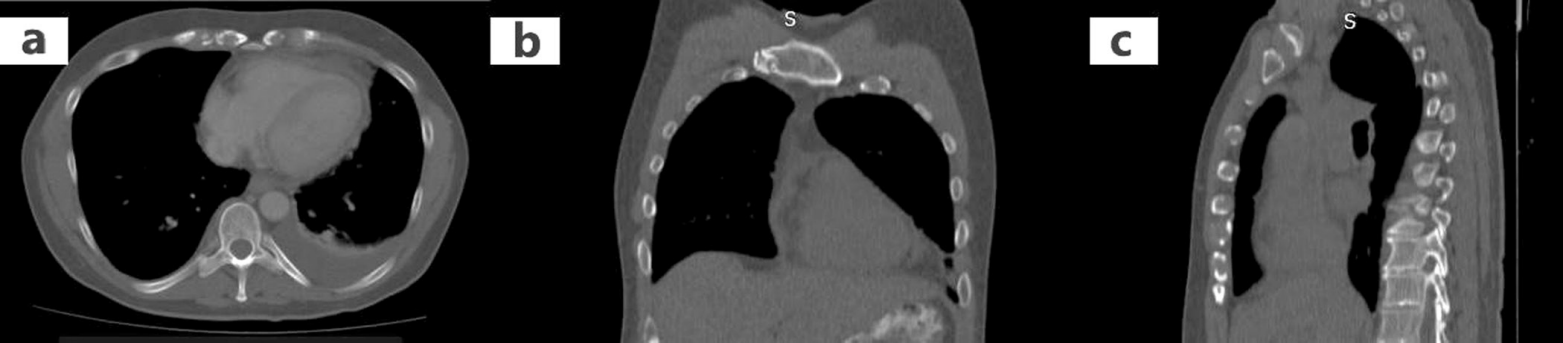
Chest computed tomography (CT) revealing a pericardial effusion and a small left pleural effusion. a: Transverse section; b: Coronal section; c: Sagittal section.
